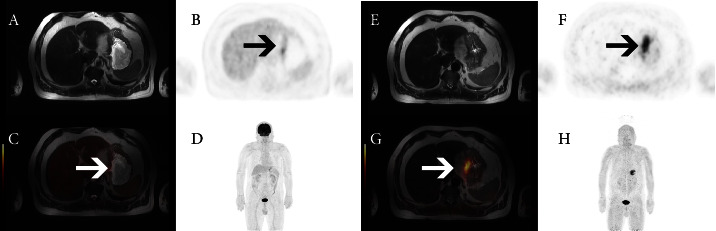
A 64-year-old male patient with gastric cancer diagnosed as gastric fundus tumor by gastroscopy. FDG-PET MRI showed a slight increase in FDG metabolism, with an SUVmax of 3.74 and no increase in metabolism in perigastric lymph nodes (A-D). FAPI-PET/MRI showed obvious thickening of the cardia, gastric fundus and lesser curvature of the gastric body, and an abnormal increase in FAPI uptake (arrow), with an SUV_max_ of 11.2 (E-H). Small lymph node shadows were detected behind the lesser curvature of the stomach and the pancreas, and there was no FAPI uptake.
